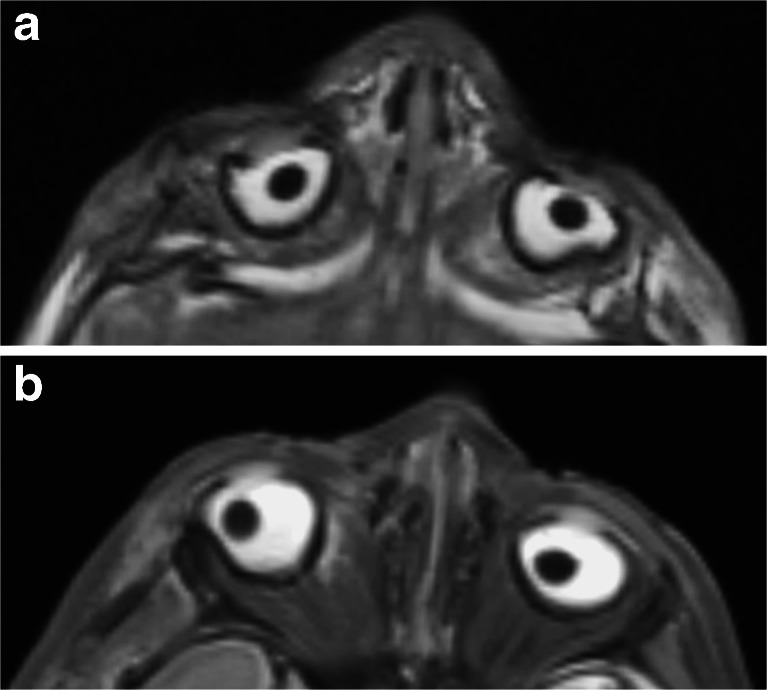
Ocular changes. Two examples of axial T2-weighted postmortem magnetic resonance imaging images from late gestation fetuses show collapse of the globes (a), with dislocation of the lens (b). These are normal postmortem changes that occur in the majority of cases, due to lack of fluid in the globe, and may be mistaken for pathology. However, lens dislocation does not always occur (see Fig. 10)
